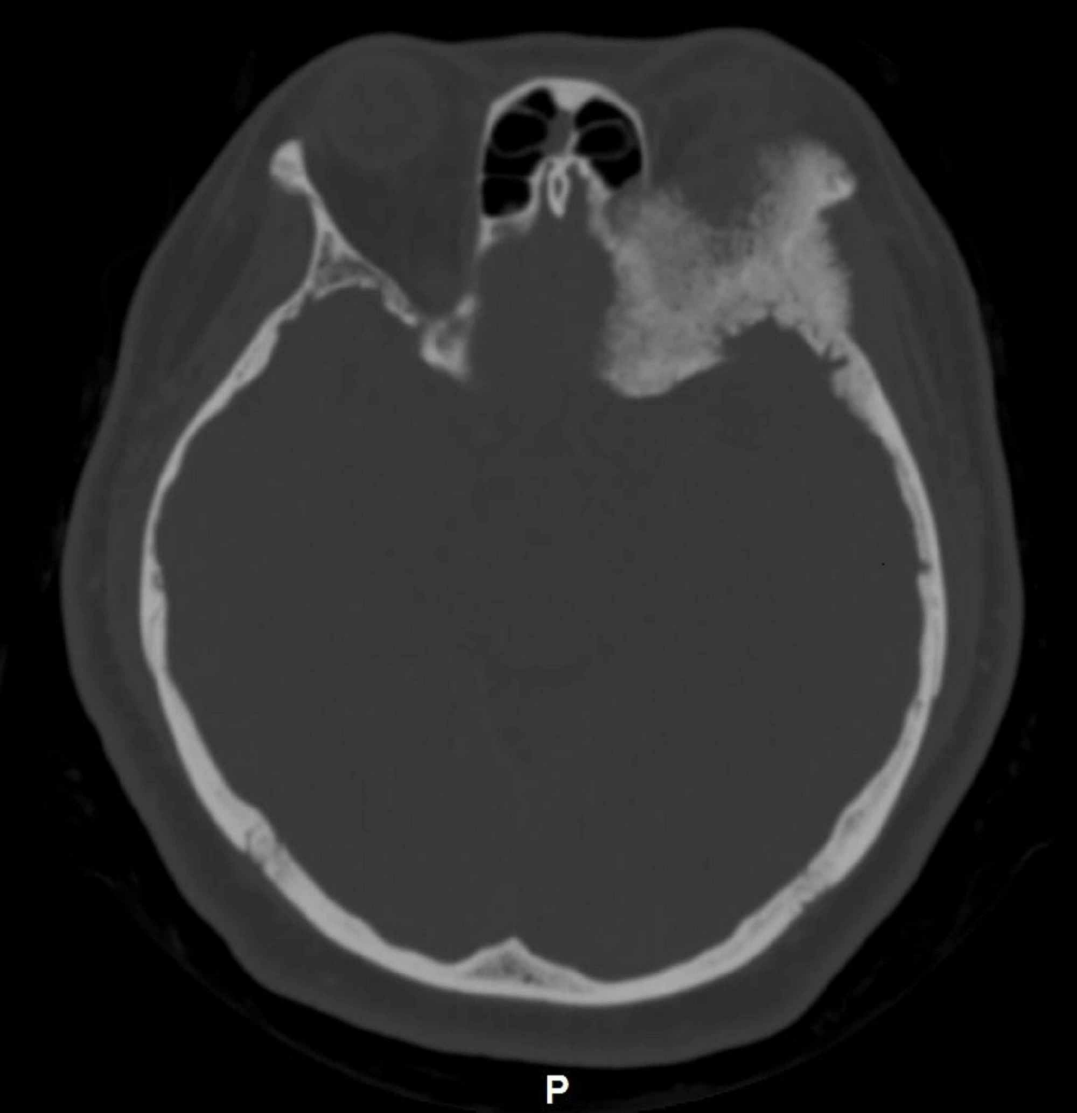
CT brain showing expansile osteoblastic lesion with irregular surface involving left greater wing of sphenoid and left zygomatic arch.
